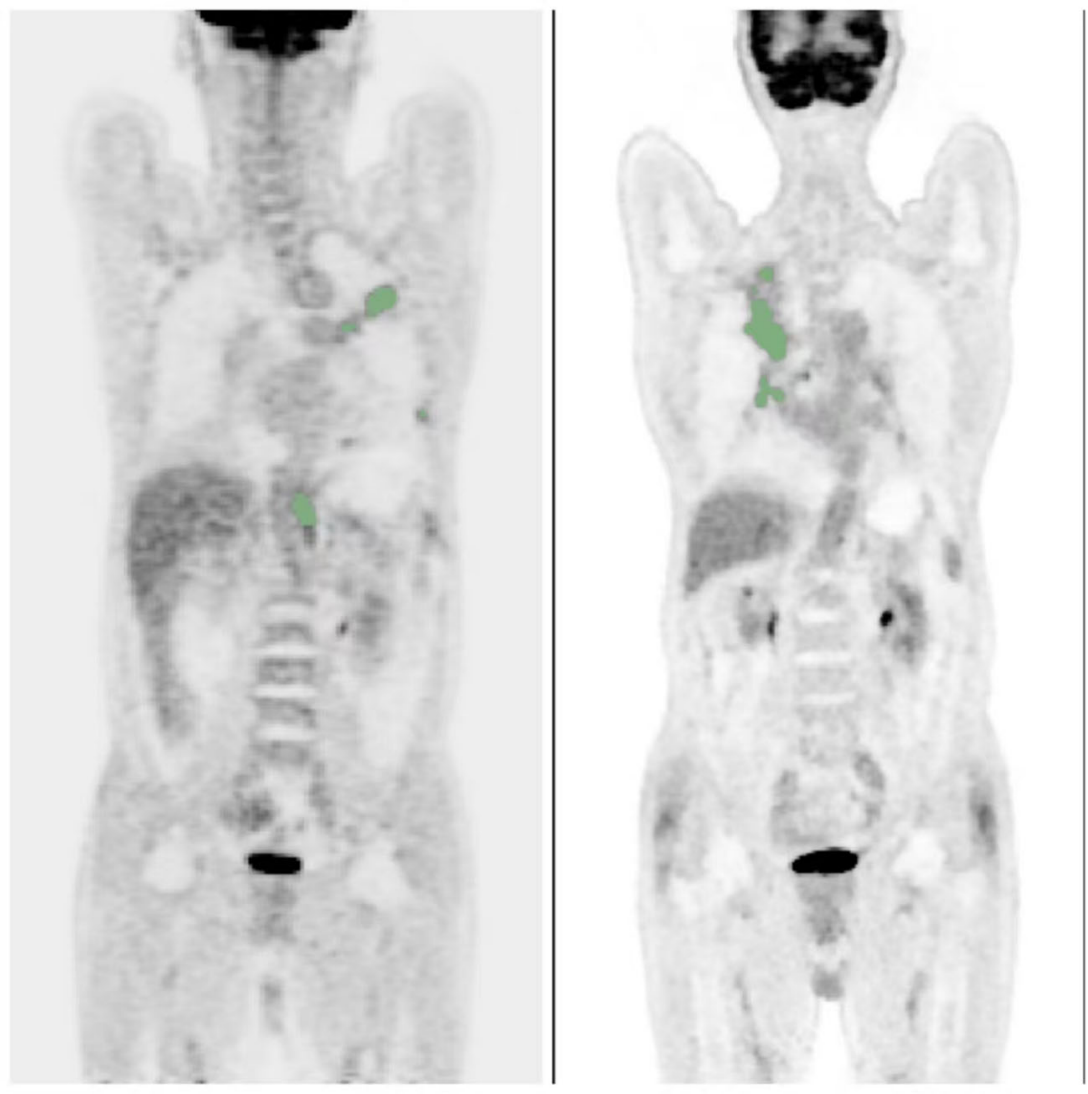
Delineation of whole-body metabolic tumor volume. NOS: Maximum intensity projection (MIP) images of an ^18^F-FDG PET/CT scan in a patient with NSCLC. The volumetric regions of interest encompassing all metastatic lesions were automatically delineated using a fixed SUV threshold of 2.5, and then manually adjusted by physicians. The total volume of these VOIs represents the MTVwb.
